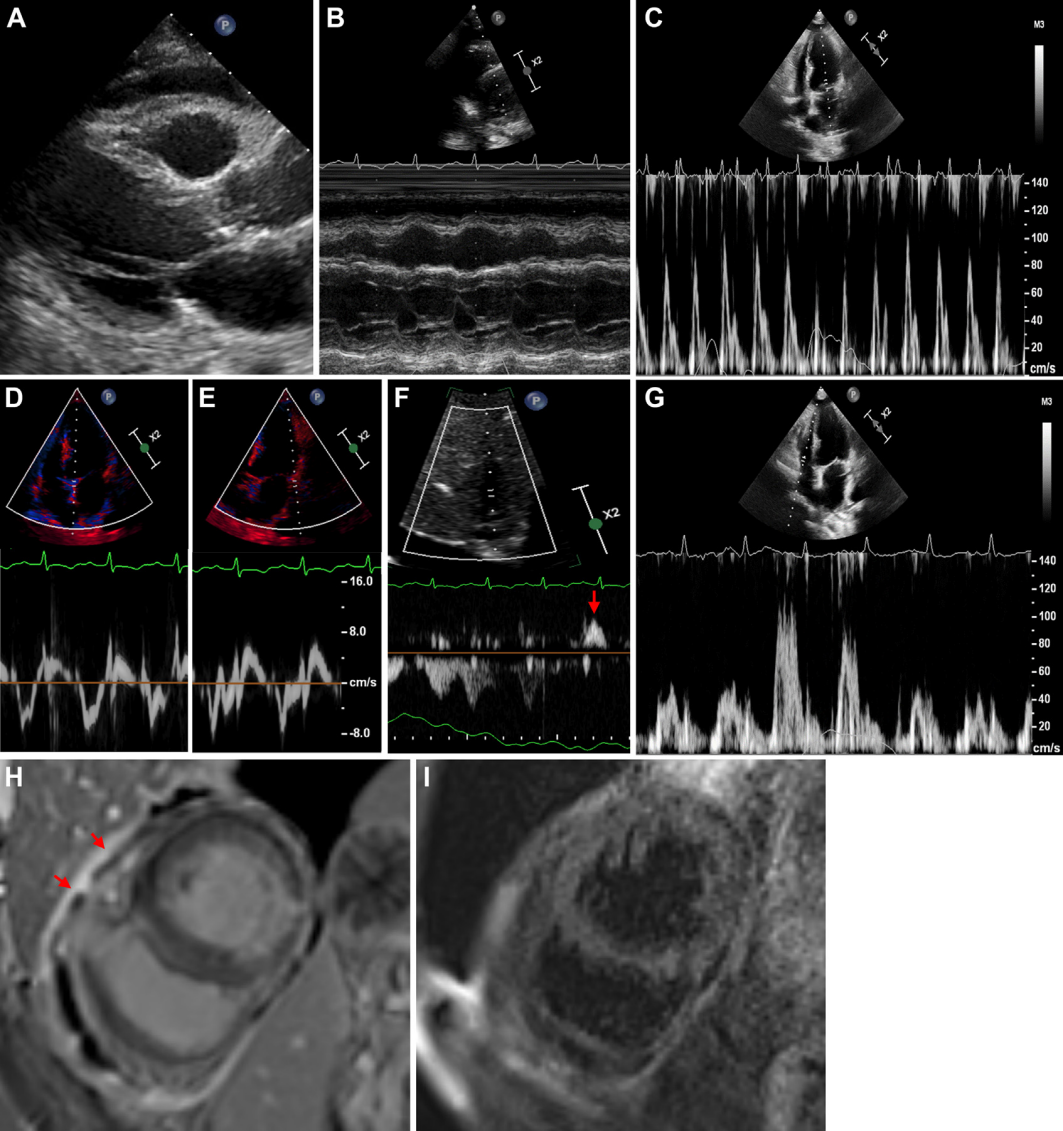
Imaging Findings of Patient 3 (A) Transthoracic echocardiography parasternal long-axis view displaying mild-to-moderate pericardial effusion adjacent to the right ventricular free wall at end diastole. (B) M mode of the parasternal short axis demonstrates significant respirophasic septal shift. (C) Mitral and tricuspid (G) inflow velocity demonstrates significant respiratory variation (41% and 59%, respectively). (D and E) Pulsed wave tissue Doppler of the mitral annulus medially (D) and laterally (E) both 8 cm/s. (F) Pulsed wave Doppler sampling of the hepatic veins demonstrating end-diastolic expiratory flow reversal (red arrow). (H) Cardiac magnetic resonance, 14 days after admission, displays pericardial thickening and delayed gadolinium enhancement on phase sensitive inversion recovery sequences (red arrows). (I) T2 black blood sequences demonstrate increased signal of the pericardial layers consistent with pericardial edema.
